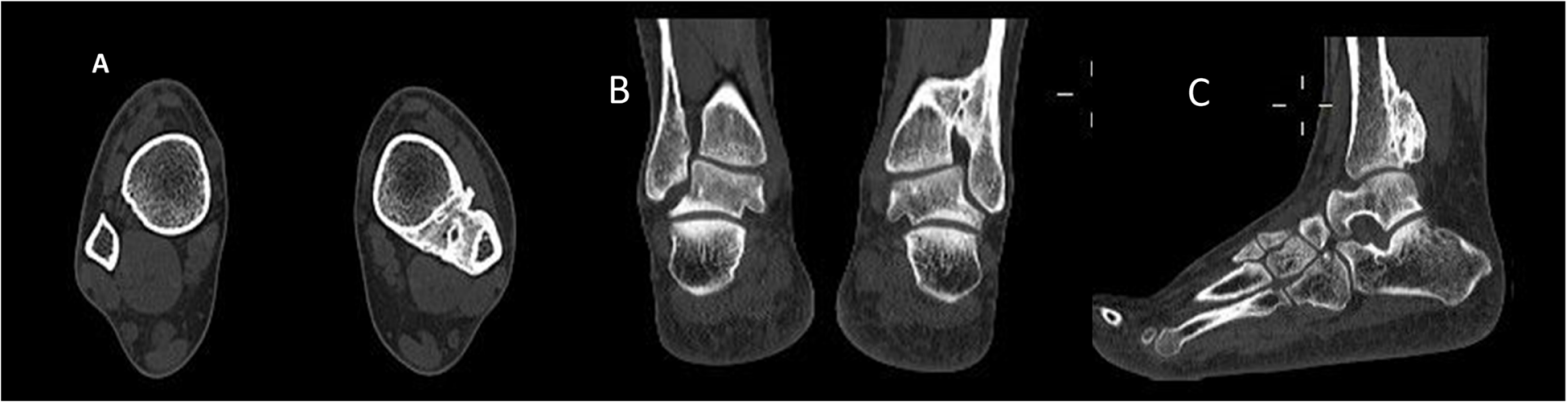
a to c 20 weeks following injury. Axial (a), coronal (b), and sagittal (c) computed tomography images of the patient’s ankle joint. Synostosis formation is evident
